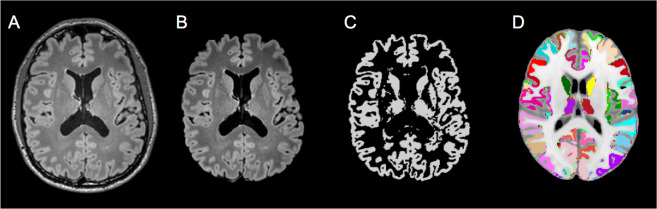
Overview of gray matter volumetry. FLAIR images of each individual (A) were reoriented and brain-extracted (B). The brain was segmented into gray matter (C), white matter and cerebrospinal fluid. Each individuals binarized gray matter map was warped onto the Automatic Anatomical Labeling atlas in MNI space (D) using linear and non-linear registration.
